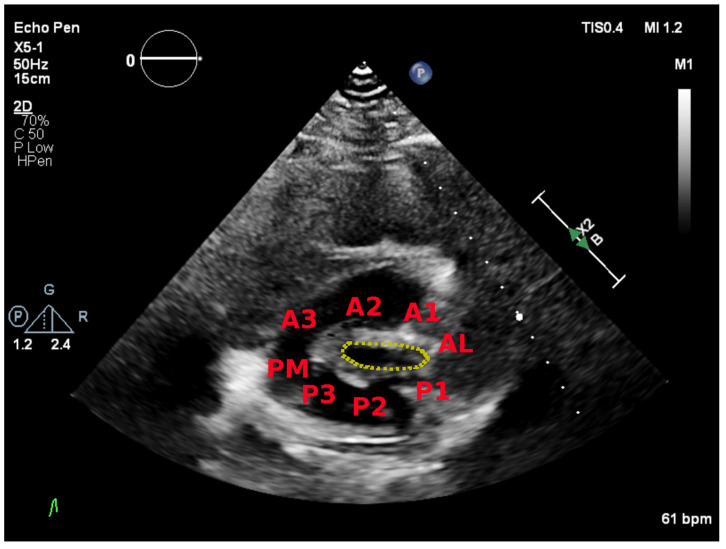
Parasternal short-axis view showing the posteromedial (PM) and anterolateral (AL) commissures and the mitral scallops.
